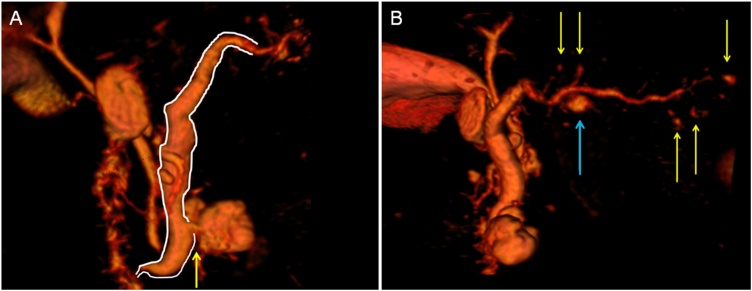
3D MRI A: MD-IPMN in the area marked by the white line and BD-IPMN in the pancreatic head (yellow arrow) B: Several BD-IPMNs located across the entire gland (yellow arrows), with a maximum diameter of 7.5 mm in the proximal pancreatic body (blue arrow). 3D Slicer Program - version 4.5 - USA [5].gr2
